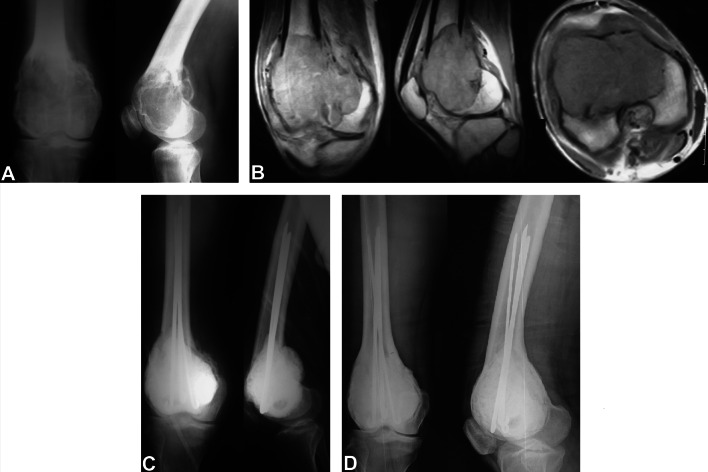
A 28-year-old male with giant cell tumor and pathologic fracture of the distal femur treated with curettage and cementation with fixation using rush pins. A Preoperative AP and lateral X-rays. B Preoperative coronal, sagittal, and axial MRI cuts. C Immediate postoperative AP and lateral X-rays. D Ten-year follow-up AP and lateral X-rays with KL grade 3 arthritic changes
